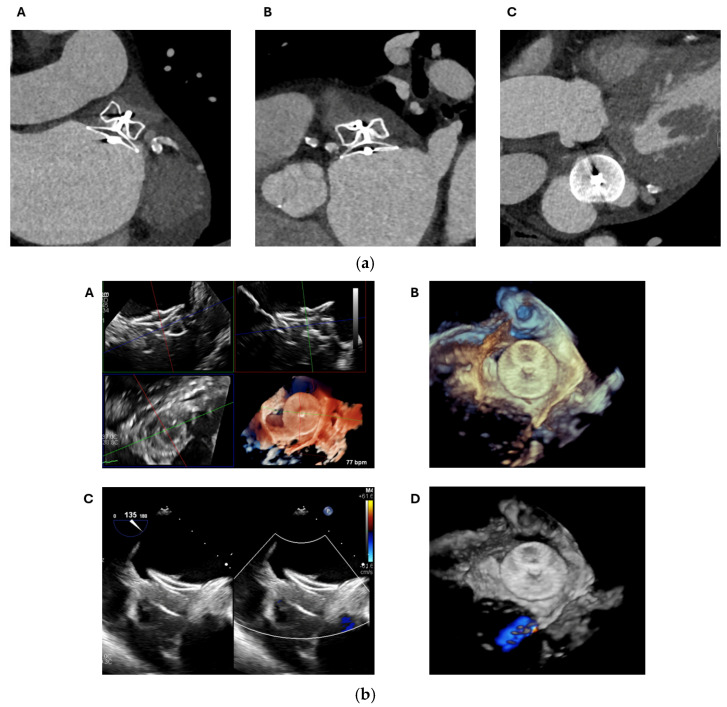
(a) A 71-year-old gentleman imaged with cardiac CT 8 weeks post LAAO with an Amplatzer Amulet device. The 3D multiplanar reformat shows a well-positioned device with no evidence of embolization, as seen on the two orthogonal views (A,B) as well as the short-axis view (C), PDL or DRT. (b) The same patient was also imaged with TOE. This confirmed a well-positioned device on 3D multiplanar reformat (A) and 3D zoom (B), with no evidence of embolization or DRT. 2D colour doppler with 135° view shown here (C) and 3D zoom with colour doppler (D) showed no evidence of PDL. LAAO: Left atrial appendage occlusion, TOE: Transoesophageal echocardiogram, 2D: Two-dimensional, 3D: Three-dimensional, CT: Computed tomography, PDL: Peri-device leak, DRT: Device related thrombus.
